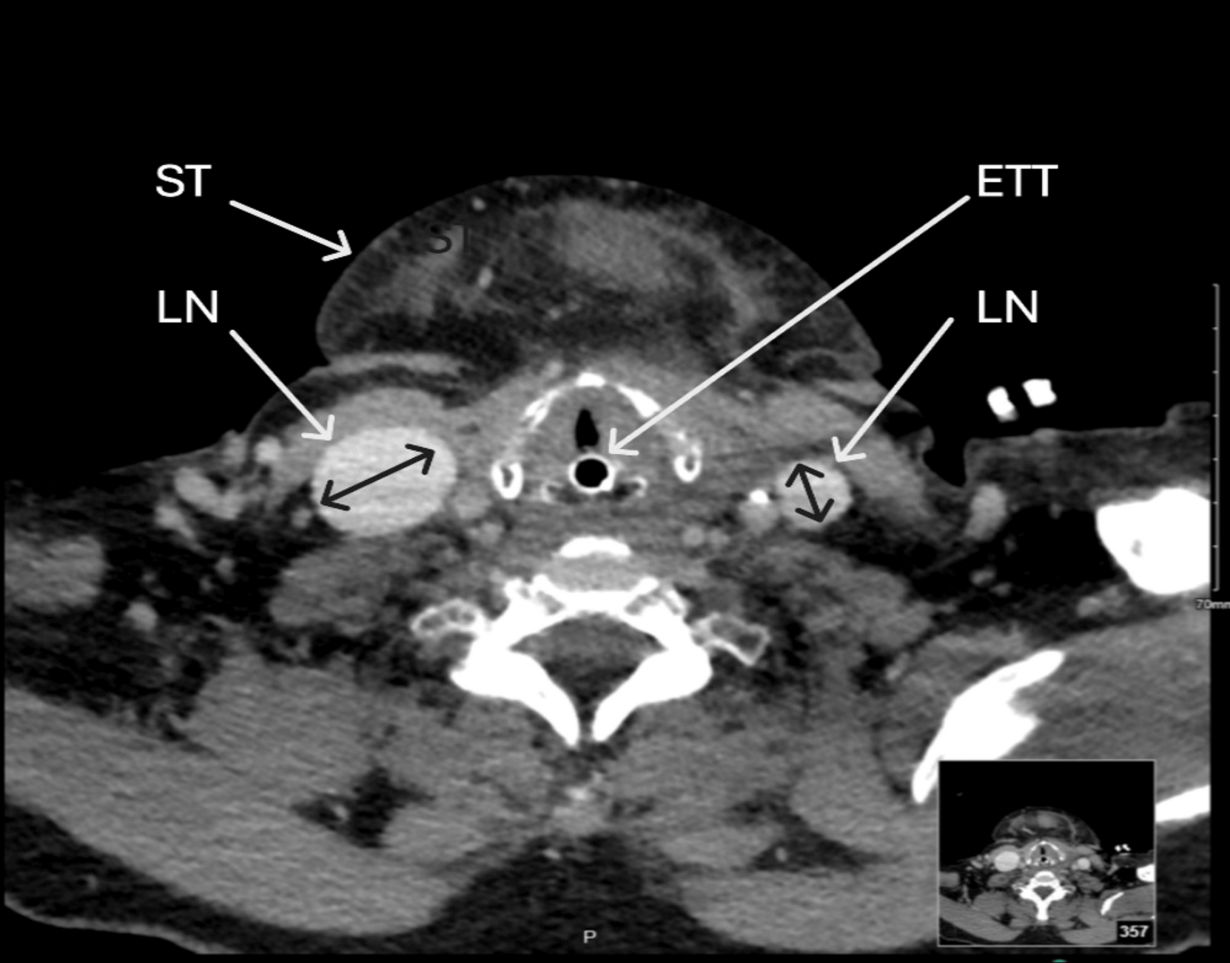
Computed tomography of the head and neck (axial view) Endotracheal tube (ETT) in situ; dental implant reflection noted. There is significant bilateral submandibular lymphadenopathy (LN) with extensive submental lymphadenopathy and soft tissue (ST) edema. An associated abscess cannot be excluded. Edema is also seen at the base of the tongue. Specialist review is required.
